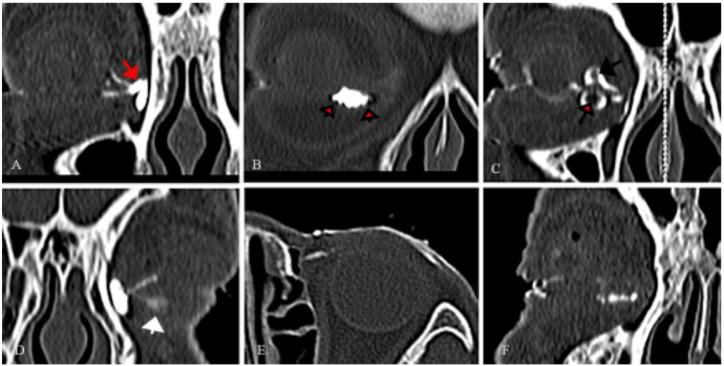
CT-DCG imaging features of primary canaliculitis. (A) The dilation of the common canaliculus (red arrow). (B) Canalicular dilation towards the temporal side with filling defects in close proximity to the canalicular lumen wall (short black arrow). (C) Both the upper and lower canaliculi showed canalicular dilation and central radiolucency with marked surrounding enhancement (long black arrow). (D) Temporal dilation of the lower canaliculus, accompanied by small filling defects (white arrow). (E) Raggedness of the canalicular lumen wall. (F) A beading appearance of the lower canaliculus.
